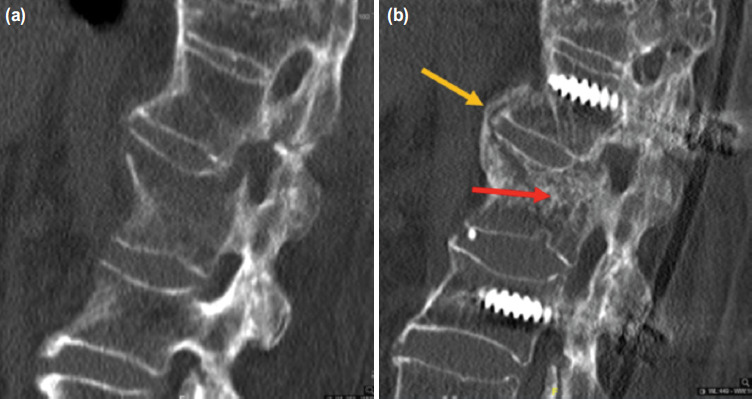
(a) CT scans showed acute hyperextension fracture evidenced by cortical breakage extending from the anterior vertebral border to the posterior vertebral border. (b) Four months postoperatively, fracture union was achieved evidenced by bridging trabeculae across the fracture site within the vertebral body (red arrow) and formation of syndesmophytes across 2 vertebral levels (yellow arrow).
